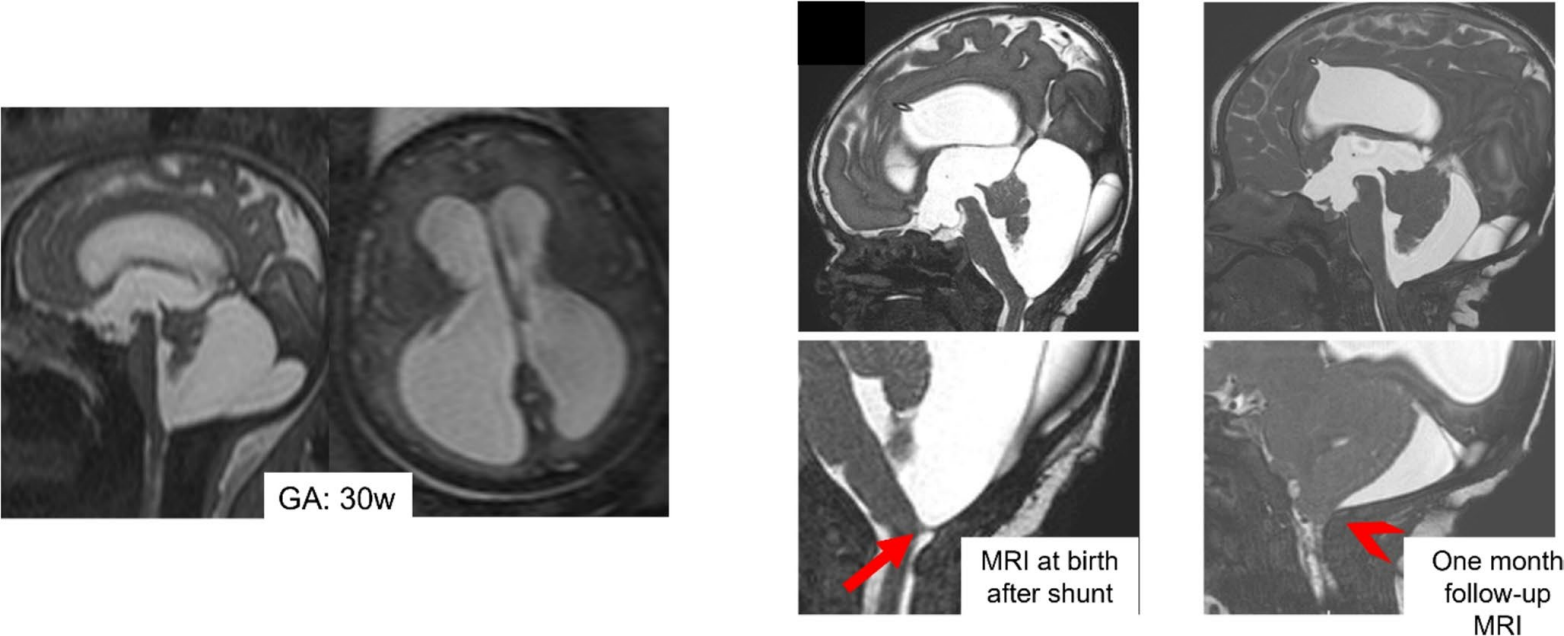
Sagittal and axial single-shot fast spin-echo T2w MR images of a 30-week fetus show obstructive hydrocephalus due to membranous obstruction of IV ventricle outlet. At birth, a shunt is placed with an almost complete return to the normal morphology of the PCF after one month
